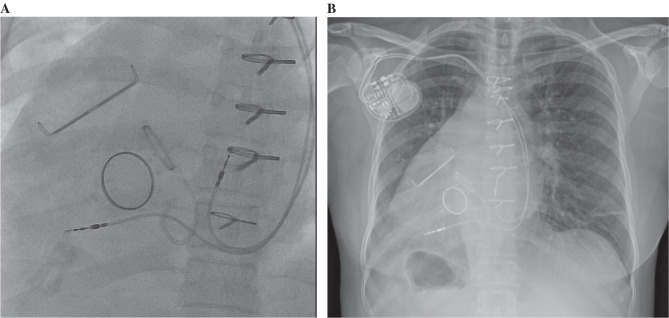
Dextrocardia with a prominent gastric bubble underneath the right hemidiaphragm is demonstrated under fluoroscopy in a 30° left anterior oblique cranial view (A) and chest radiograph in the anteroposterior view taken after the insertion of a dual-chamber pacemaker (B).
